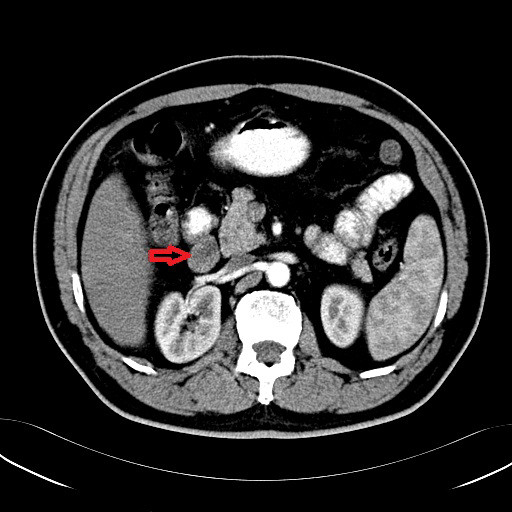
Computed tomography scan of the abdomen reveals a space-occupying lesion originating from the descending part of the duodenum (red arrow).
